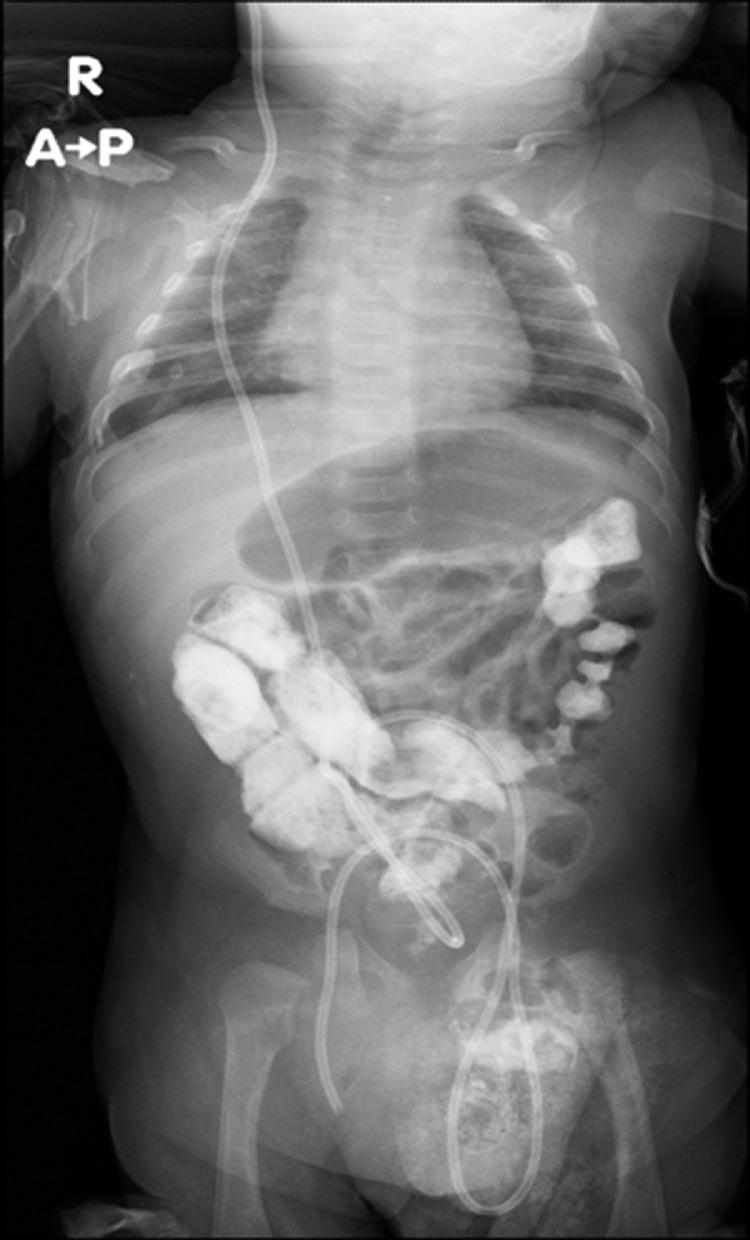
Follow-up abdominal radiograph, unchanged.
